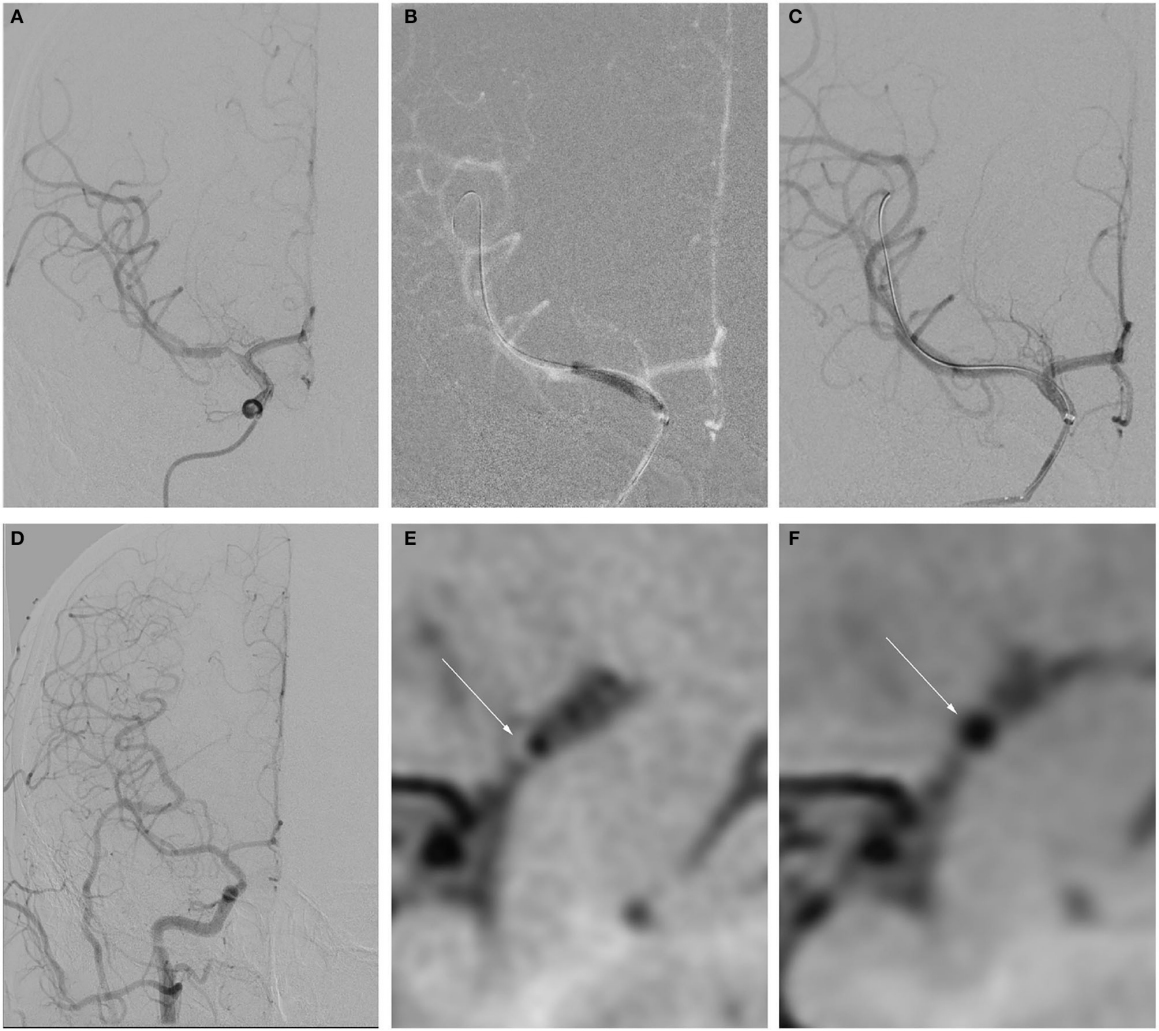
Example of drug-coated balloon (DCB) dilation and follow-up. (A–F) Angiography and high-resolution MRI (HRMRI) results of the DCB dilatation during the procedure and follow-up. (A) Severe right middle cerebral artery (MCA) stenosis. (B) DCB dilatation after predilatation. (C) The angiographic result after the procedure. (D) Angiographic result at 3.3-month follow-up. (E) Focal eccentric plaque identified at target vessel wall [arrow in (E)] in high-resolution T1-weighted imaging sequence. (F) No plaque or wall thickening was identified on high-resolution T1-weighted imaging sequences at 3.3-month follow-up.
